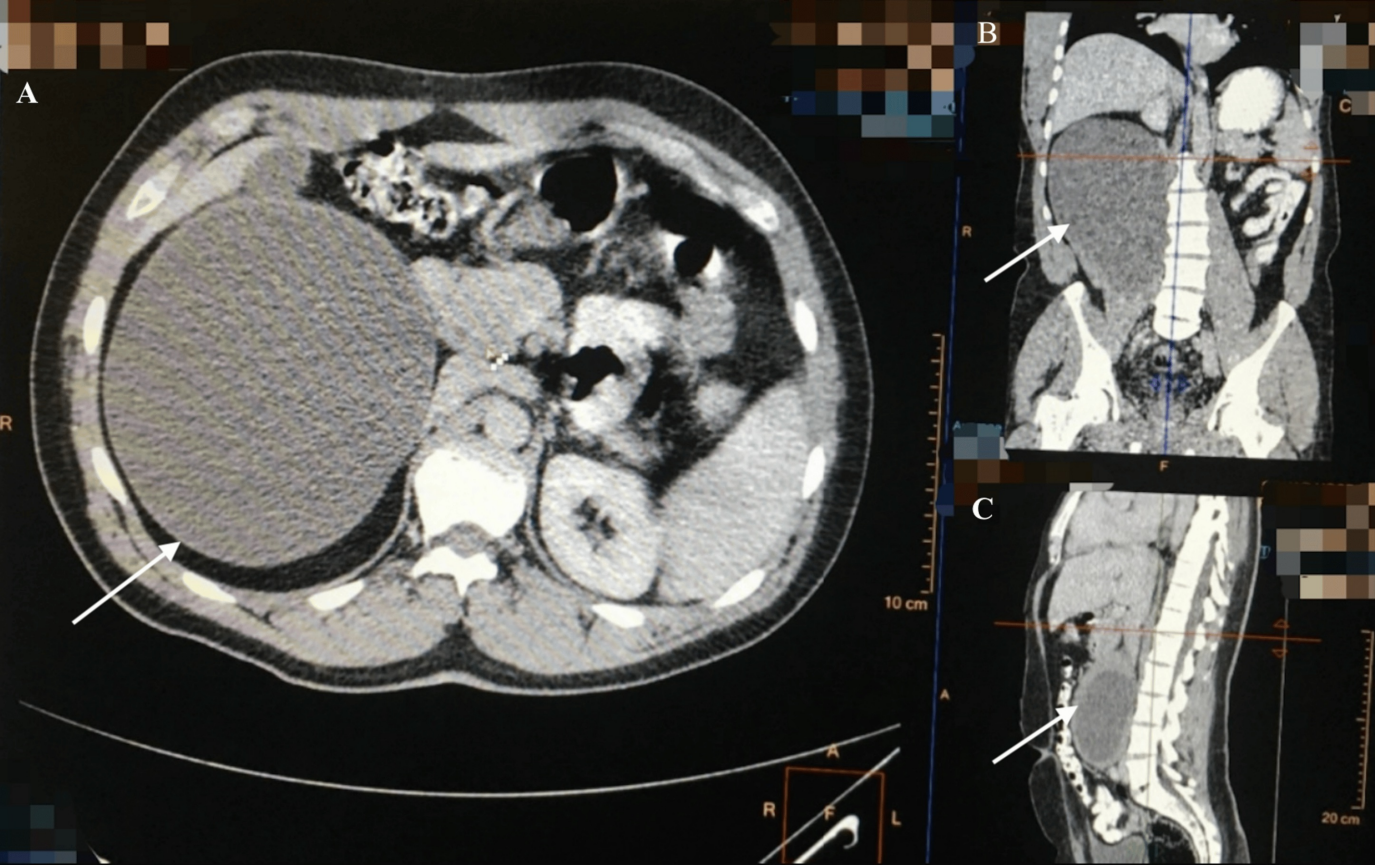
CT image demonstrating a large, well-defined cystic lesion (20 x 14 cm) within the right psoas muscle (arrows) (A) Axial view. (B) Coronal view. (C) Sagittal view. CT: computed tomography
